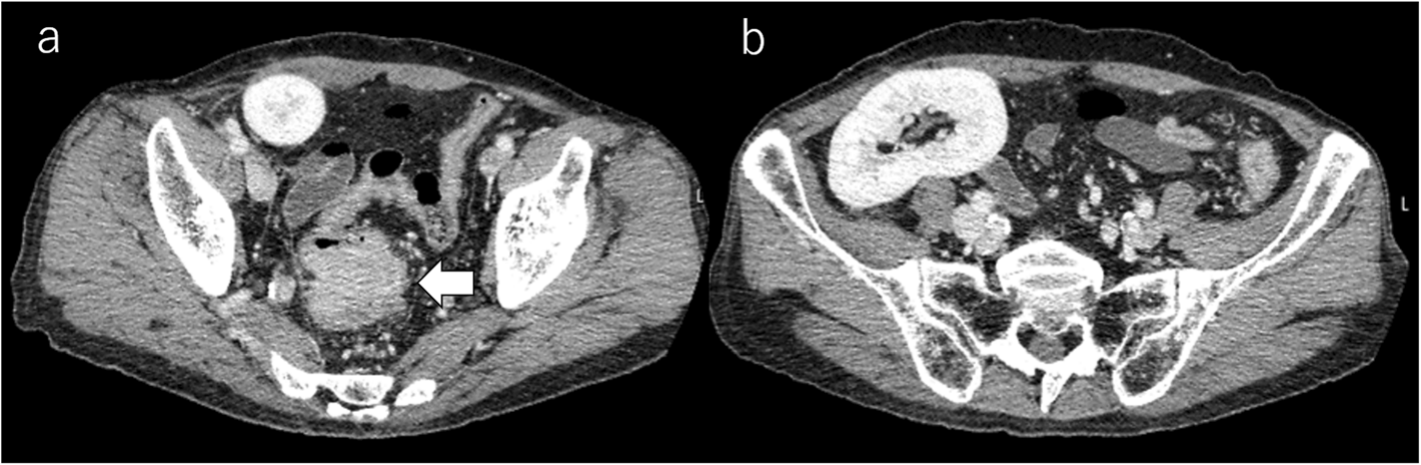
A CT scan revealed a circumferential mass with irregular surface and contrast effect and wall thickening at the rectosigmoid colon (a, arrow) and the transplanted kidney located in the right pelvic cavity (b)
